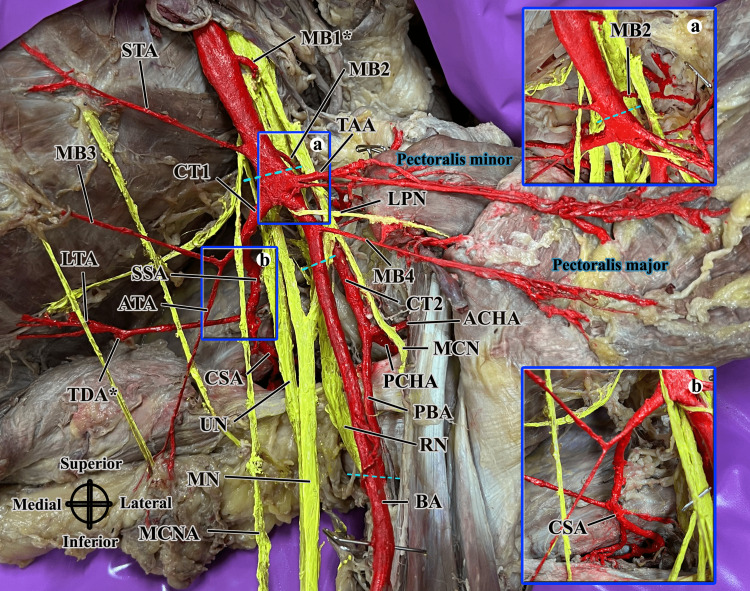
Branching variations and anteromedial course of the left AA in relation to the brachial plexus. Asterisks (*) indicate branches that were severed during the dissection. Nerve structures are painted yellow, arterial structures painted red, muscles labeled in blue font, and dashed blue lines indicate the boundaries of the parts of the AA. Directional indication is provided in the lower left corner. (a) A close-up of MB2, an accessory branch to the subscapularis muscle. (b) A close-up of the CSA. AA: axillary artery; ACHA: anterior circumflex humeral artery; ATA: alar thoracic artery; BA: brachial artery; CSA: circumflex scapular artery; CT1; common trunk 1; CT 2: common trunk 2; LPN: lateral pectoral nerve; LTA: lateral thoracic artery; MB1: muscular branch 1, seen coursing to the clavicular region; MB2: muscular branch 2, seen piercing the subscapularis muscle; MB3: muscular branch 3, an accessory lateral thoracic branch; MB4: muscular branch 4, an accessory pectoral branch; MCN: musculocutaneous nerve; MCNA: medial cutaneous nerve of the arm; MN: median nerve; PBA: profunda brachii artery; PCHA: posterior circumflex humeral artery; RN: radial nerve; SSA: subscapular artery; STA: superior thoracic artery; TAA: thoracoacromial artery; TDA: thoracodorsal artery; UN: ulnar nerve.
